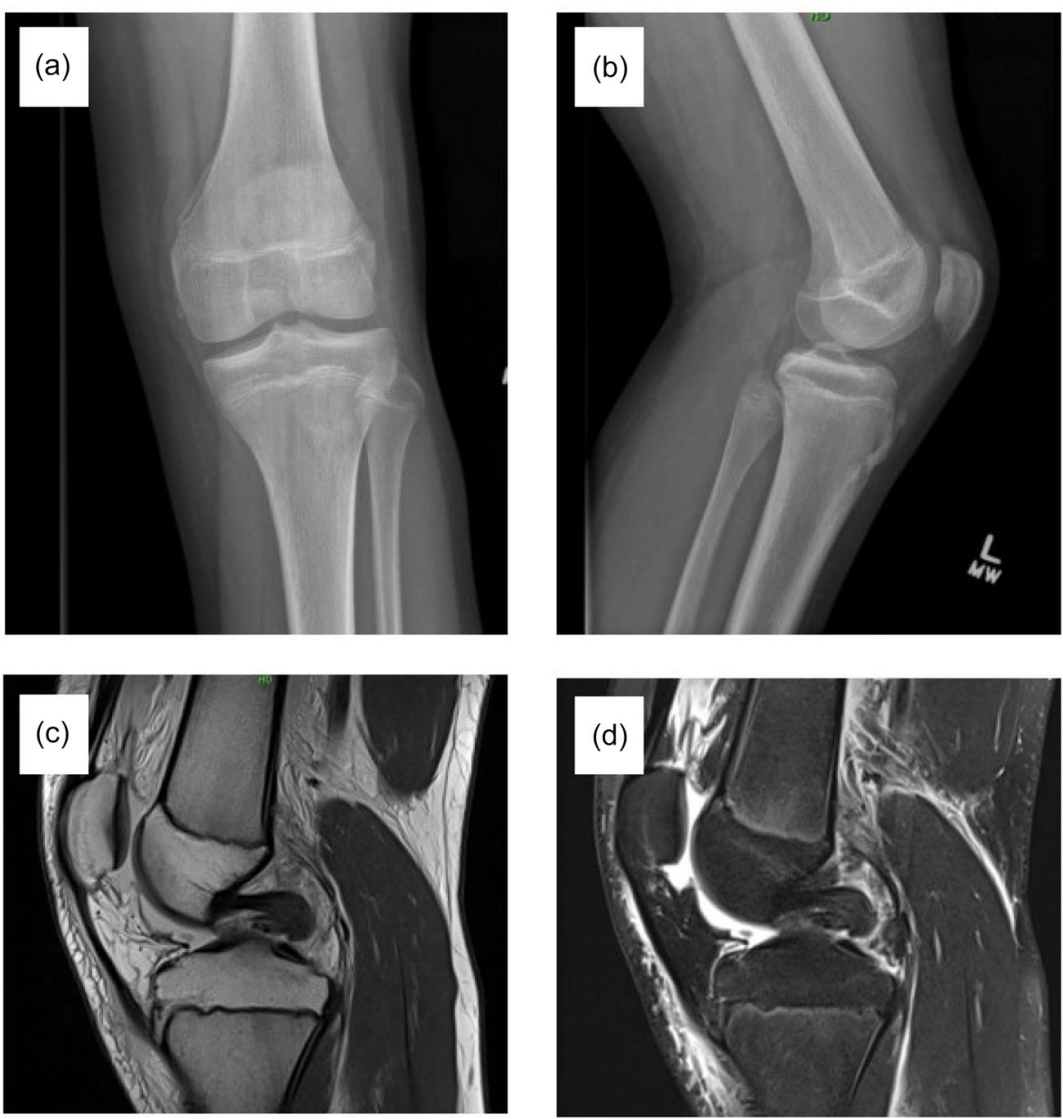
Radiographic imaging of a 14‐year‐old male who sustained a complete PCL tear while water tubing. The patient successfully returned to sports after 7 months of physical therapy and sustained no recurrent injuries at latest follow‐up. (a) Anterior/posterior view x‐ray. (b) Lateral view x‐ray. (c) Sagittal view T1 weighted MRI. (d) Sagittal view T2 weighted MRI. MRI, magnetic resonance imaging; PCL, posterior cruciate ligament.
